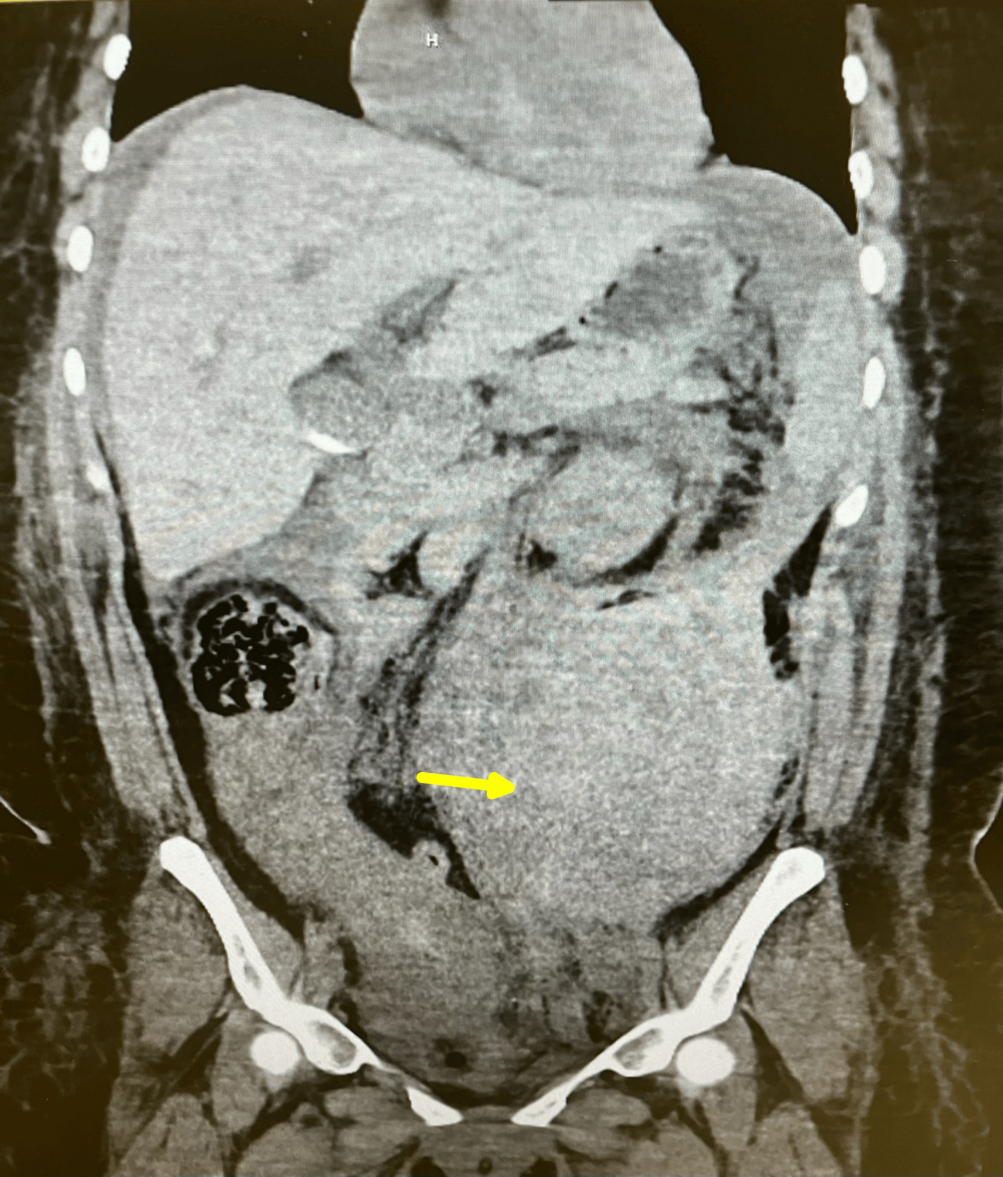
Coronal CT angiography showing a large retroperitoneal hematoma (arrow) measuring 16.6 x 13.9 x 14.5 cm with an adjacent pseudoaneurysm.
